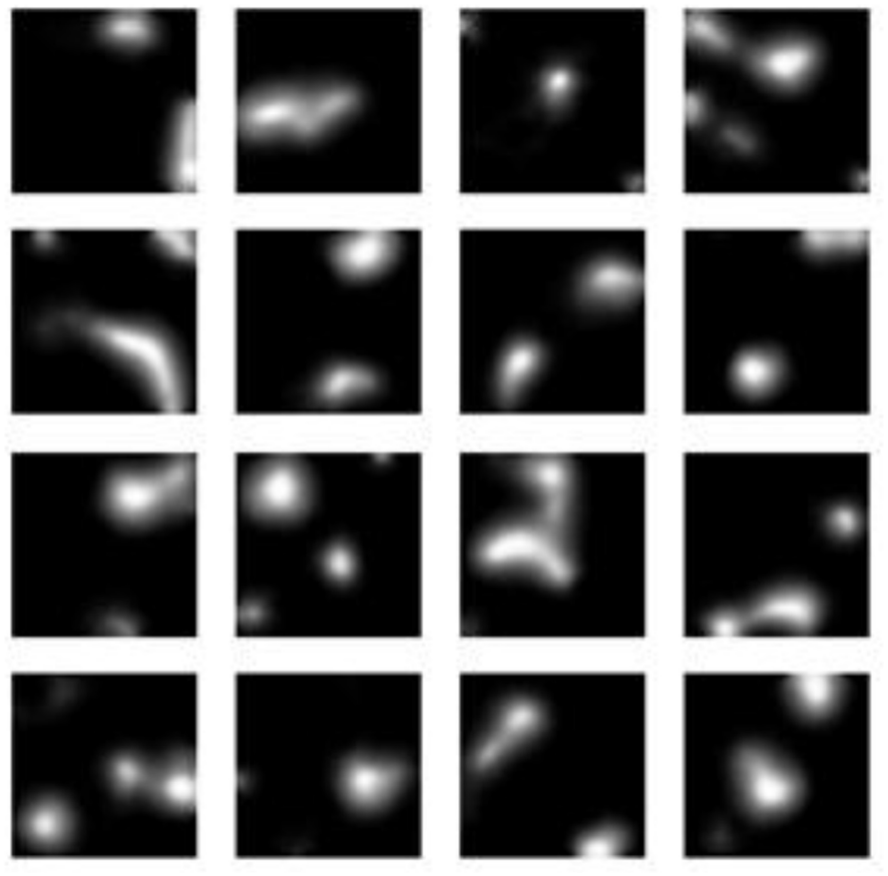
MRI generated by the mustGAN model.
